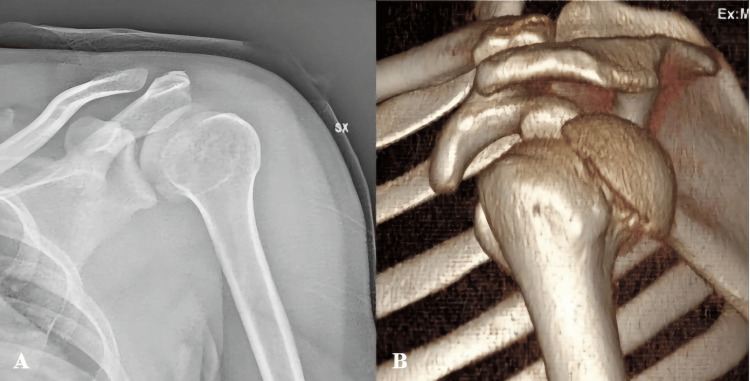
(A) Initial plain radiograph and (B) CT scan 3D reconstruction of the affected shoulder.
